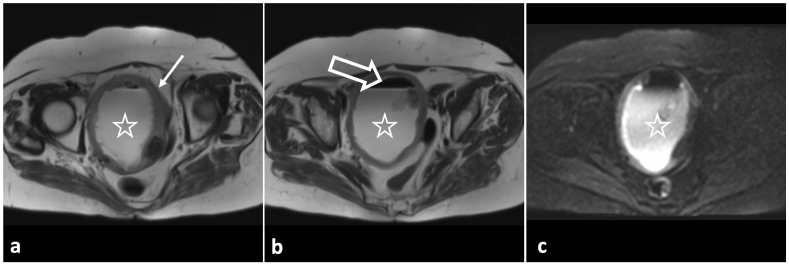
axial MR images. a; T2WI images show a thick-walled (thin arrow) cystic lesion (star). b; there is air-fluid leveling within the lesion (thick arrow). c; DWI images show diffusion restriction of the fluid component of the lesion.
